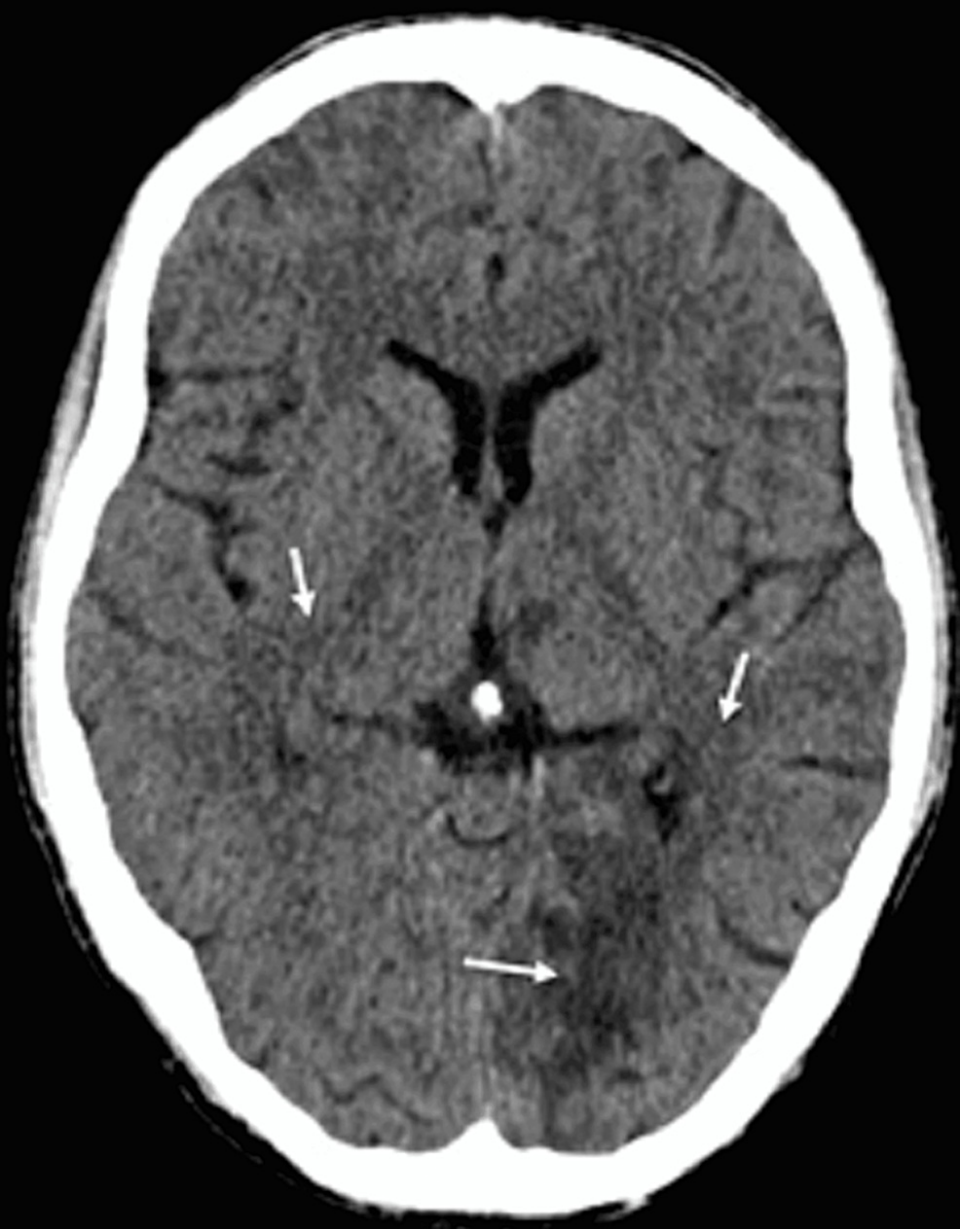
Head CT without contrast showing multifocal infarctions (white arrows)
